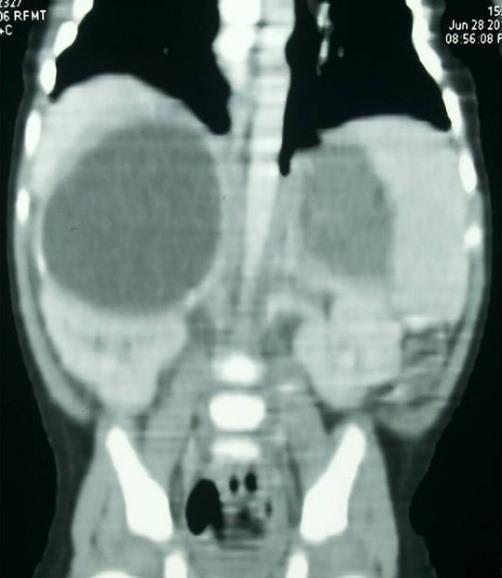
Case 2, Abdominal computed tomography image (coronal section) showing encysted collections with enhancing rims with fine internal septations in bilateral suprarenal areas, measuring 6.8 × 4.6 cm on the right side and 2.5 × 1.8 cm on the left side.
